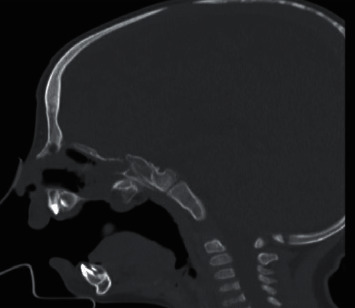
CT facial bones: cleft lip and palate with approximately 10 mm gap within the hard palate and prominent posterior bone vomer with no skull base defect.
